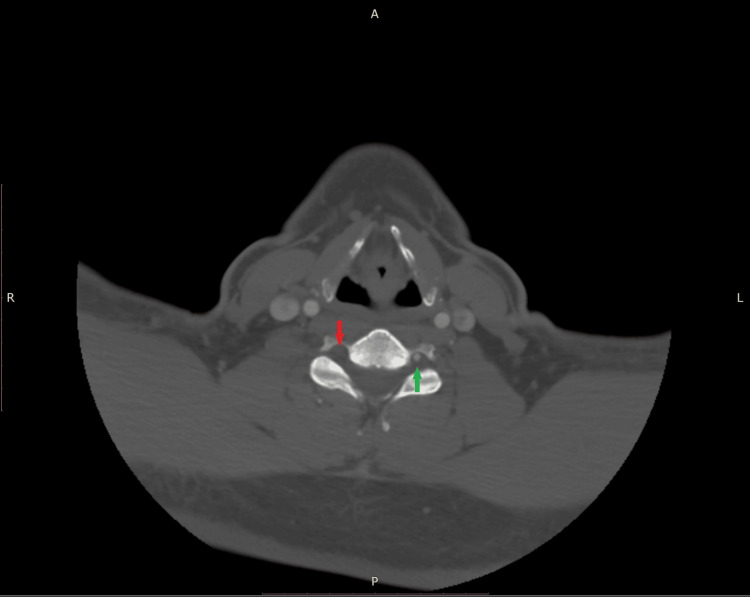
Axial view of a CT angiogram obtained on the day of presentation demonstrating no passage of contrast through the right transverse foramen (red arrow). The passage of contrast is observed through the left transverse foramen (green arrow).
